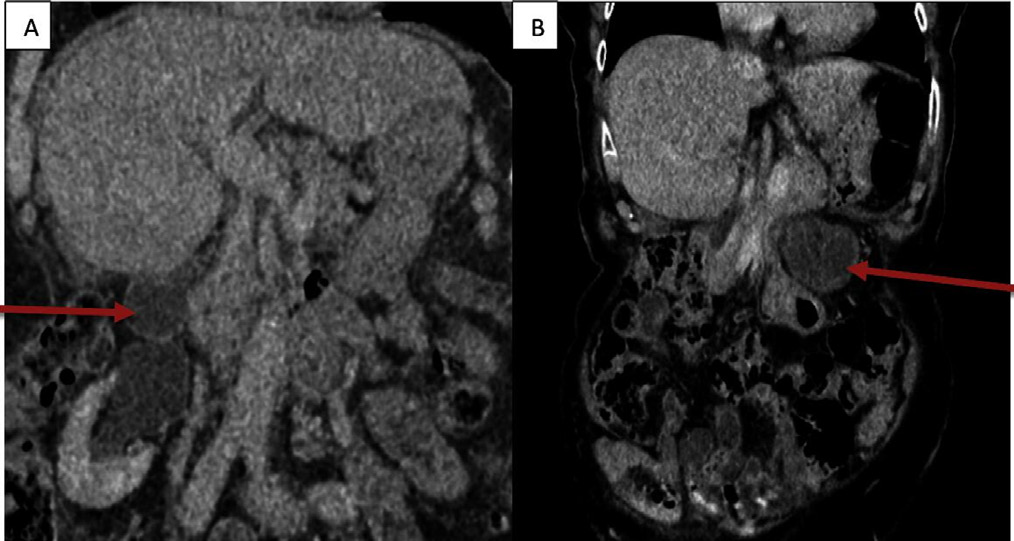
Comparison images:CT scan in 2018 and CT scan during admission (2020) A – Coronal oblique reconstruction: CT 2018 showing normal location of gall bladder Image B: CT 2020: Coronal view showing the suspected gallbladder with signs of torsion
